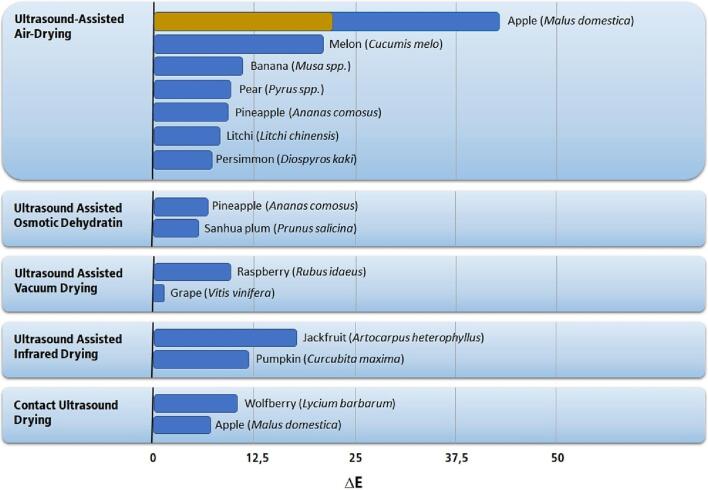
Highest changes of color in fruits by applying ultrasound technologies [24], [25], [38], [92], [95], [97], [99], [102], [103], [119], [147], [148], [158], [159], [168]. Single-colored bars indicate unique values in the literature. Double-colored bars indicate the lowest (brown) and highest (blue) values reported in the literature.
